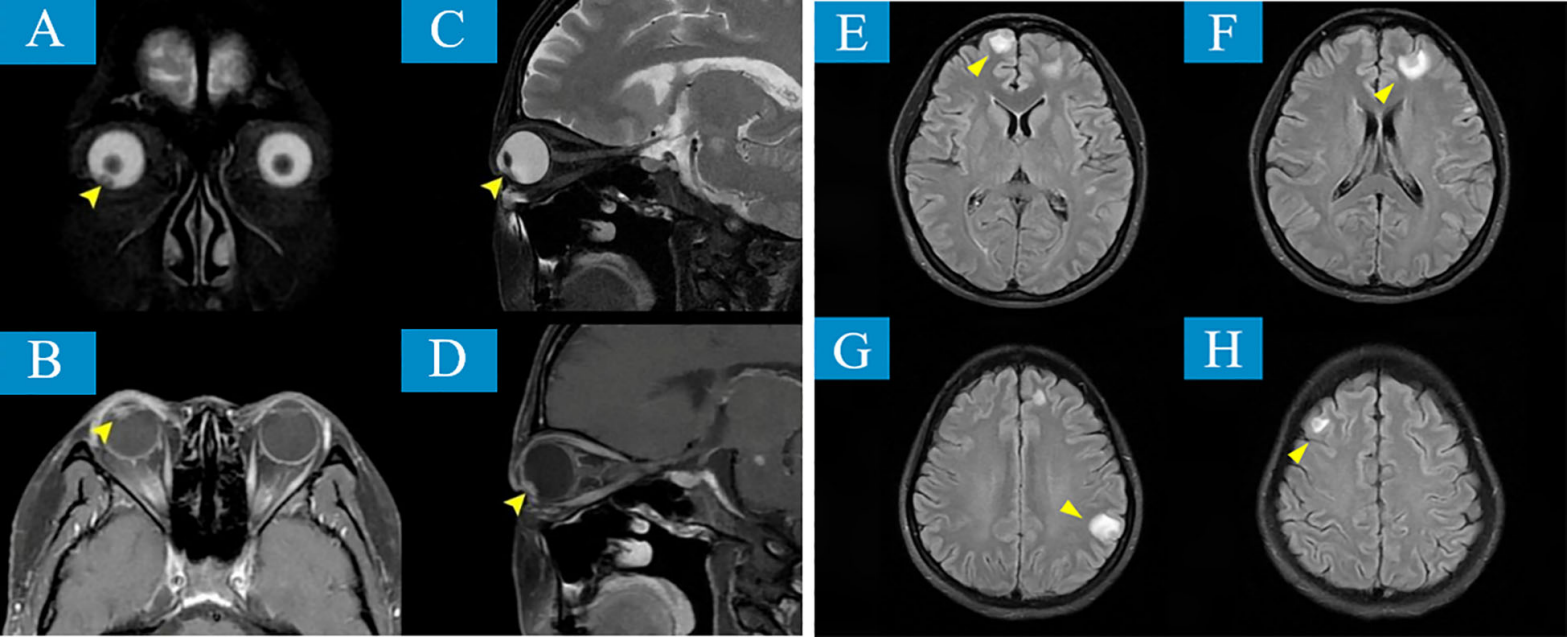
MRI reveals lesions involving the right eyeball and intracranial regions. (A, B) T2-weighted sequences (T2WI) and (C, D) T1-weighted sequences (T1WI) reveal an isointense mass, whose location is identified as the inferotemporal iris and ciliary body of the right eye based on ophthalmic anatomy (yellow arrows). In addition, multiple nodular abnormal signal intensities are observed in the left pons, bilateral cerebellar hemispheres, frontal and parietal lobes, and the left temporal lobe (yellow arrows) (E–H).
